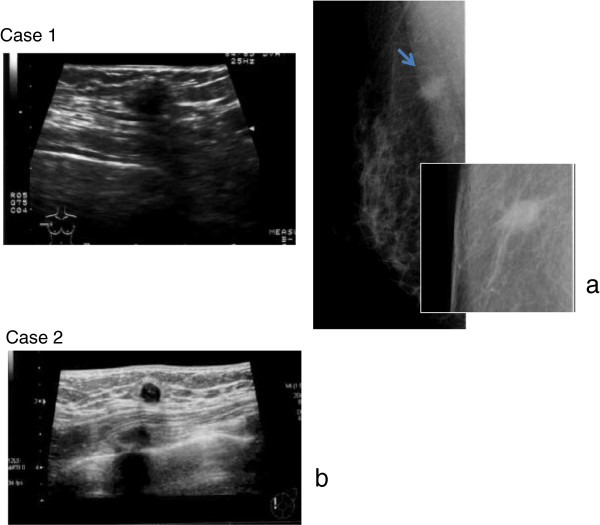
(a) Case 1. Ultrasonography revealed a tumor 8 × 9 mm in size with irregular borders and rupture of the anterior border of the mammary gland. Mammography revealed a tumor with irregular borders and spicule. (b) Case 2. Ultrasonography revealed a tumor 7 × 8 mm in size with irregular borders and rupture of the anterior border of the mammary gland.
